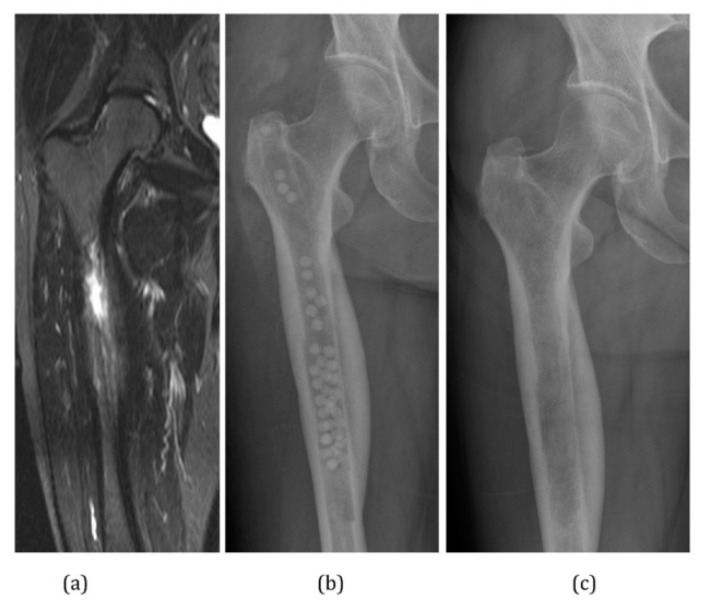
(a) MRI image demonstrating extensive medullary edema, intramedullary abscess, and cortical involucrum. (b) Infection treated by excision via medullary reaming. Dead space filled with calcium sulphate pellets loaded with gentamicin. Shown via radiograph (c) follow-up radiograph at 4 months post-operatively. Calcium sulphate pellets have dissolved completely. Reprinted from Ferguson et al. [68] following the Creative Commons Attribution (CC BY) license (http://creativecommons.org/licenses/by/4.0/) (accessed on 16 September 2021).
